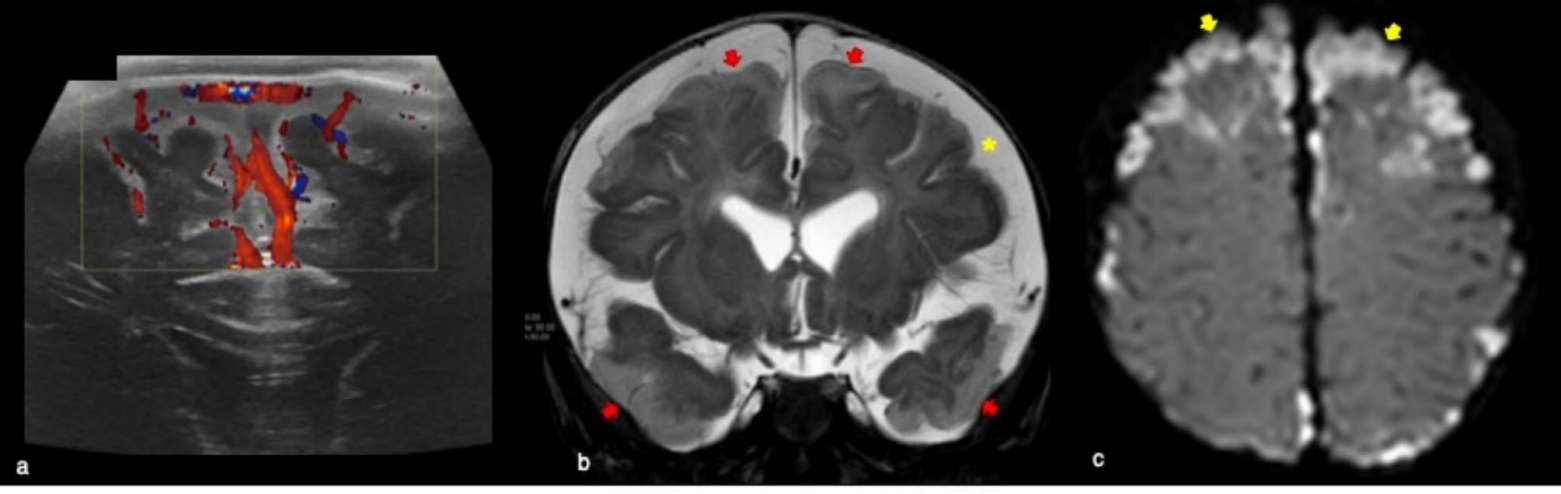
Four-month old male affected by Haemophilus Influenzae meningitis who underwent cerebral ultrasound showing dilatation of extra-axial spaces and increased vascularization on Doppler (a). MR of the same patient revealed bilateral subarachnoid empyema in the frontal, temporal and parietal areas on coronal T2 weighted (red arrows in b). Bilateral enlargement of supratentorial subarachnoid spaces was also present (yellow asterisk in b). DWI showed bilateral subarachnoid empyema and cortico-subcortical areas of ischaemia (yellow arrows in c)
